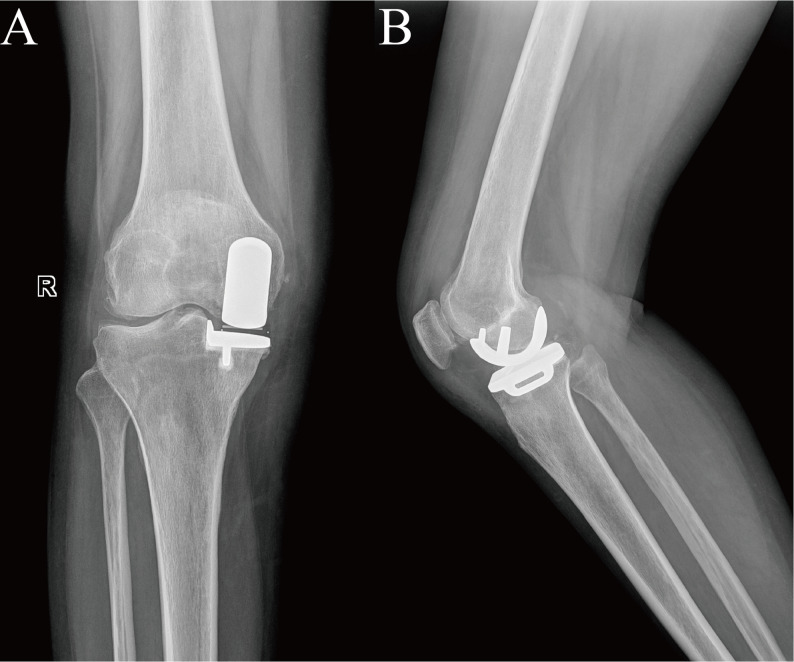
Postoperative anteroposterior and lateral radiographs of the right knee following unicompartmental knee arthroplasty. A Anteroposterior view shows the medial unicompartmental prosthesis in the right knee is well positioned with satisfactory alignment. B Lateral view demonstrates balanced flexion-extension gaps and secure fixation of the components, with no evidence of loosening or migration
